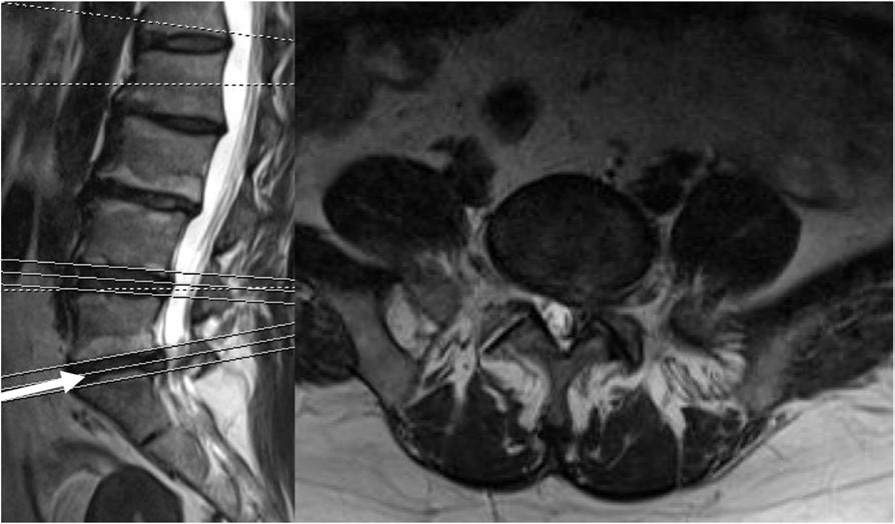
Preoperative MRI showed L5/S1 LSS caused by disc herniation and hypertrophy of the facet joint on the left side
